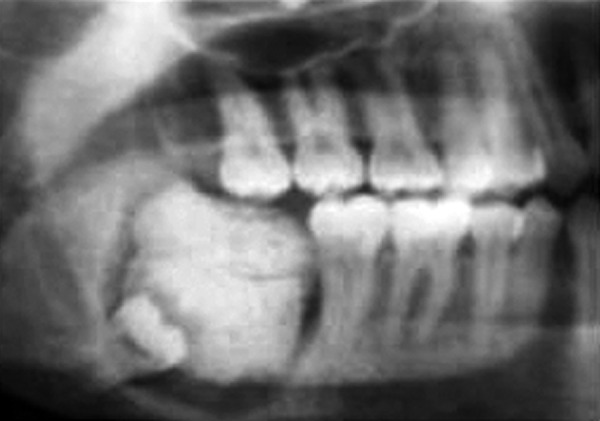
Radiograph of complex odontome
